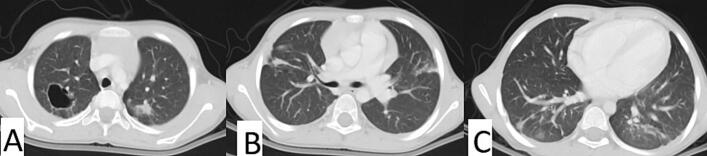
Shows axial CT images (A-C) taken at different levels of the lungs, four months post-surgery. The images reveal no recurrence of cystic lesions, with clear lung fields and normal pulmonary structures visible.
